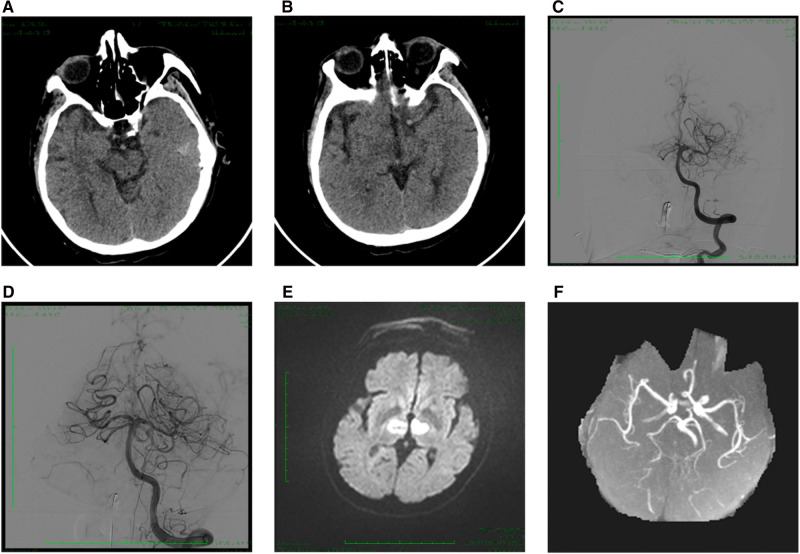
Case 1. (A,B) Baseline CT indicated pc-ASPECTS score was 9. (C) Anterior-posterior angiogram revealed an occlusion of right PPCA. (D) Post-thrombectomy angiogram indicated the mTICI score was 2b. (E) Post-procedural DWI showed acute bilateral thalamic infarction. (F) MRA showed successful recanalization of right PPCA at 48 h after procedural. PPCA, proximal posterior cerebral artery; pc-ASPECTS, posterior circulation Alberta Stroke Program Early CT Score; mRS, modified Rankin Scale.
